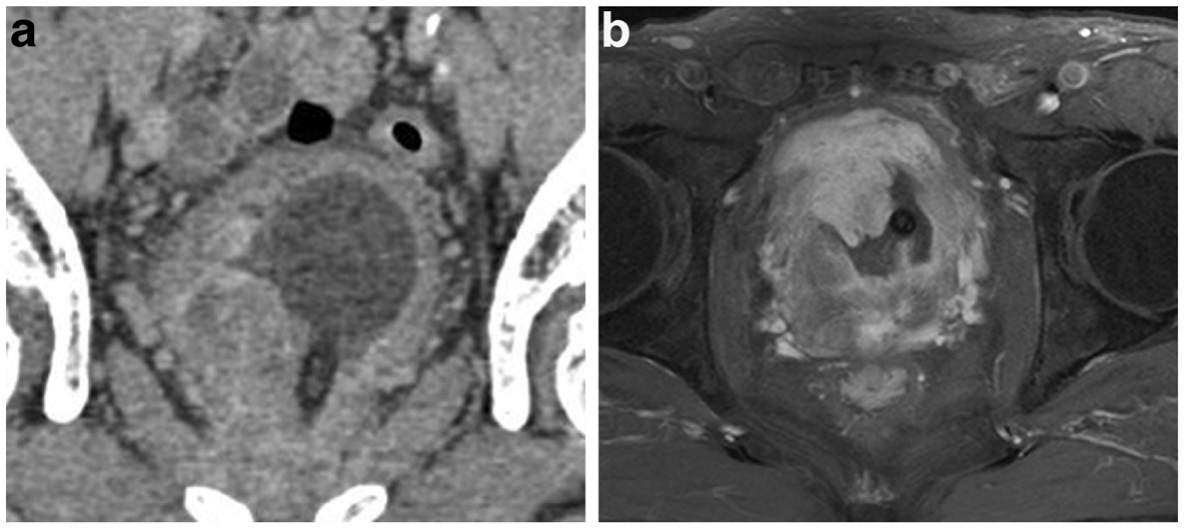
Image analysis. a) Computed tomography shows a prostatic non-homogenous mass lesion irregularly protrudingd into the bladder space. b) Gadrinium-enhanced T1 weighted magnetic resonance imaging shows a heterogeneous hyperintense mass.
